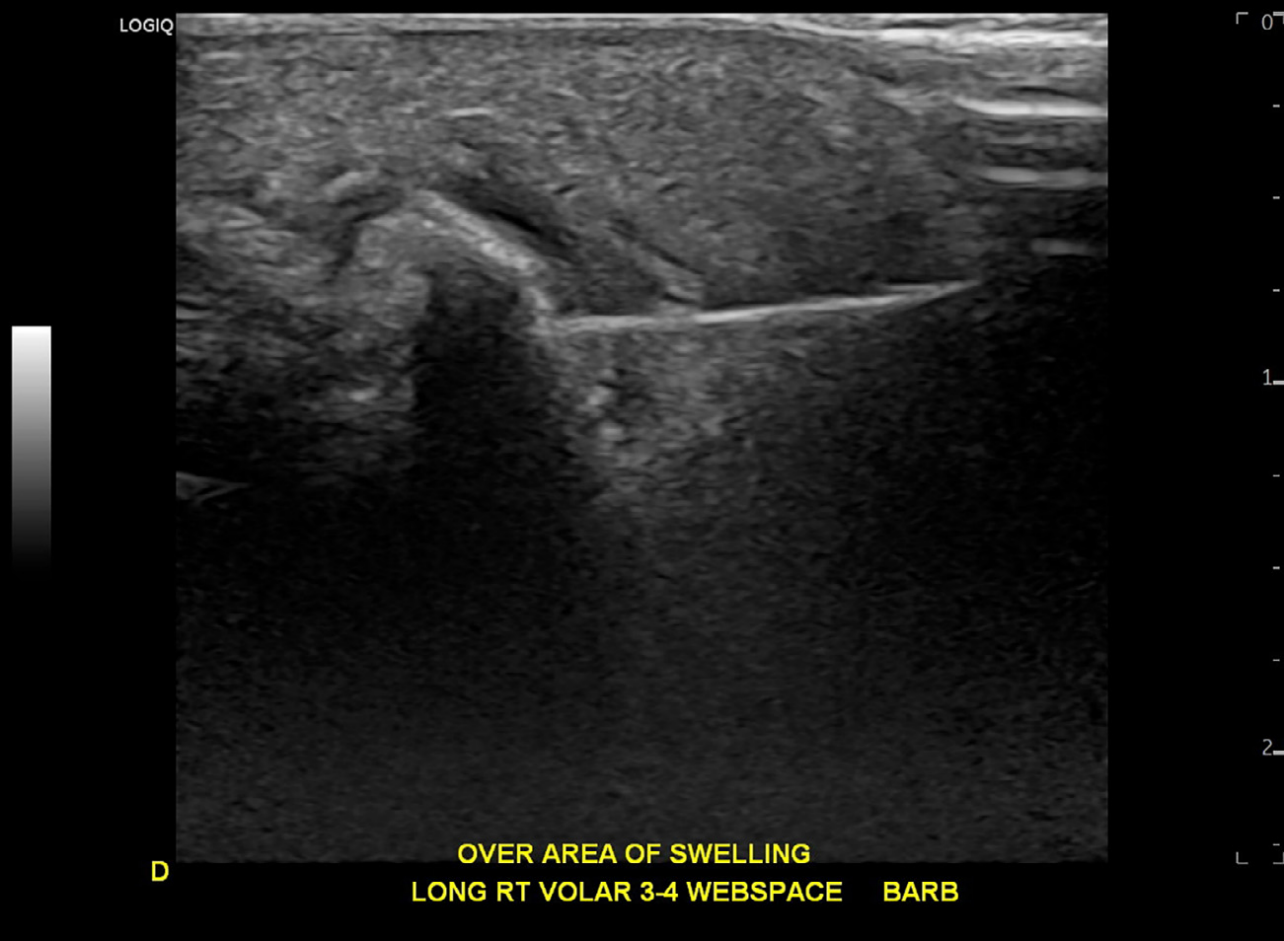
Longitudinal ultrasound image of the right hand demonstrates needle within the calcific focus. Shadowing from the calcium limits visualization of the needle tip on this single image.
